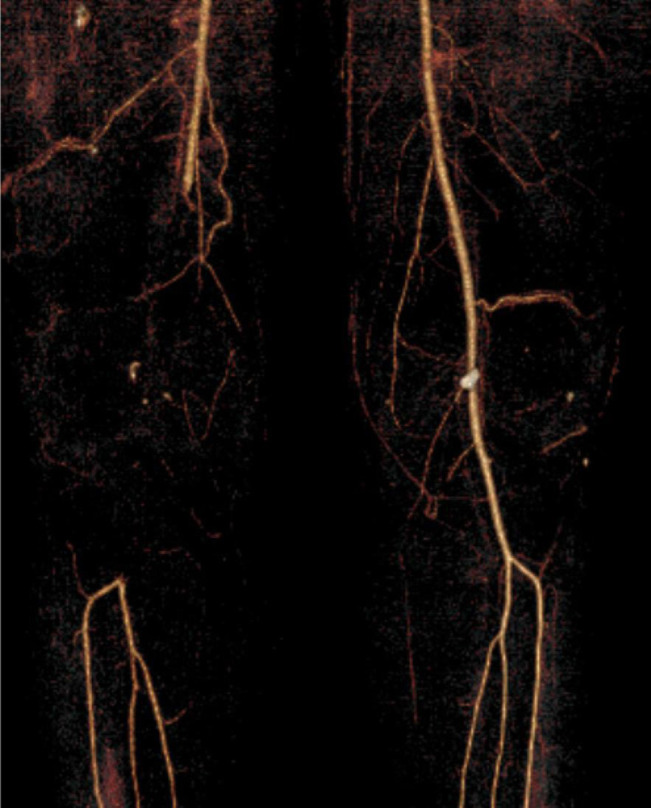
3D reconstruction of CTA demonstrating complete graft thrombosis in the right lower extremity. CT image obtained after external fixation removal and manipulation under anesthesia.
